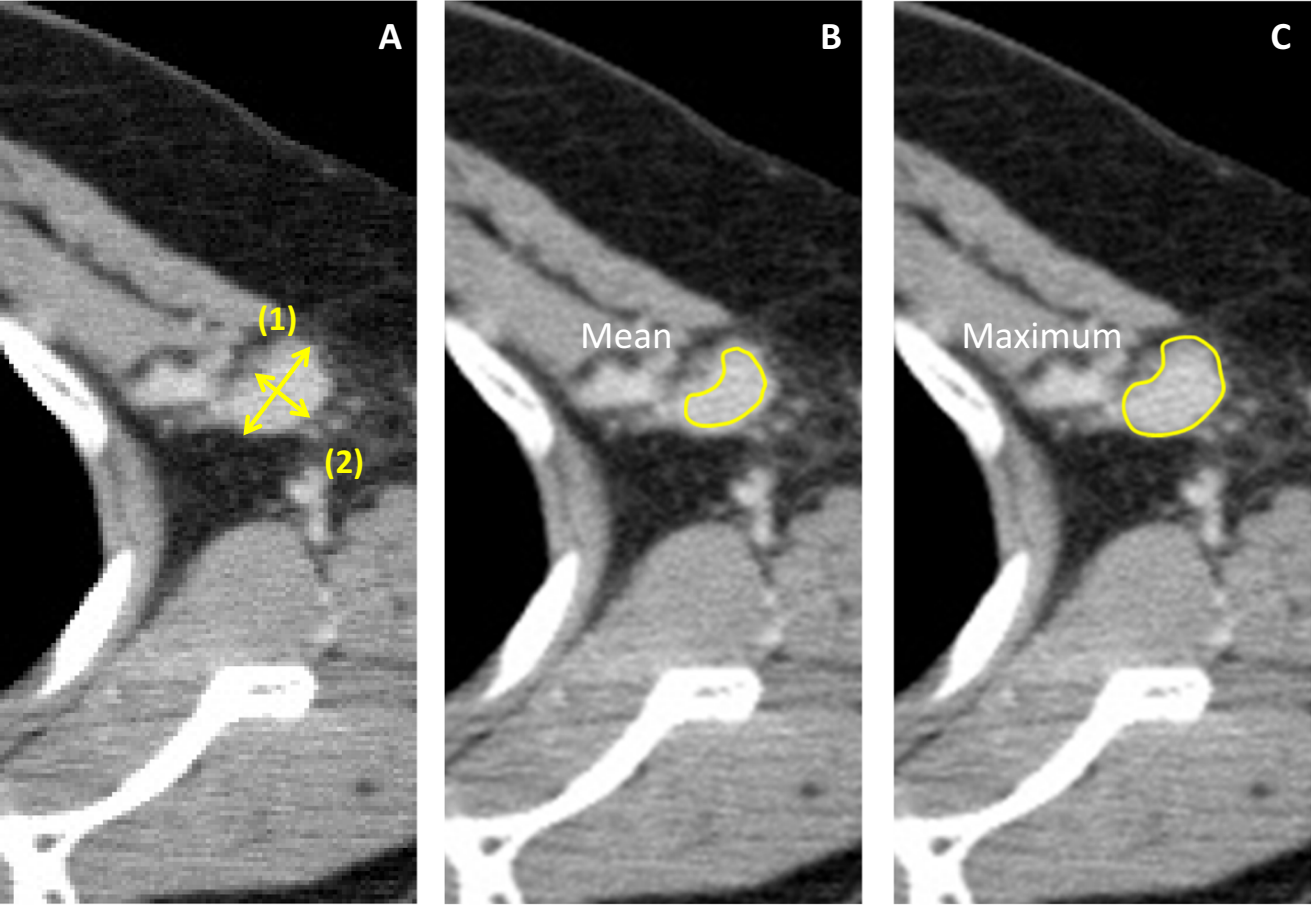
Measurement of axillary lymph nodes in a patient with invasive ductal carcinoma in the left breast. A. ALN sizes were measured in the maximum sectioned surface of the CT image with 3-mm slices: (1) Longest transverse diameter of the oval lymph node; (2) Shortest transverse diameter of the oval lymph node; B. To measure mean HU, we selected a range of interest (ROI) such that the line did not protrude from the ALN edge. C. ROI surrounded the lymph node, and the maximum HU inside of the ROI was measured. We took extra care not to include bone or blood vessels near the ROI.
